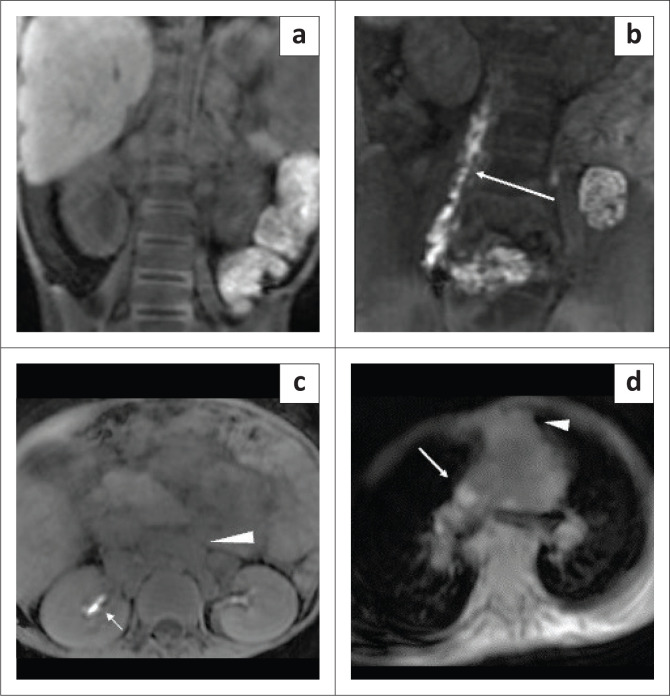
Dynamic contrast enhanced MR lymphangiography was performed in the same child for chylous ascites. (a) Coronal pre-contrast T1-weighted image of the abdomen and pelvis and (b) coronal T1-weighted image of the abdomen and pelvis at 10 min after nodal contrast injection showed dilated lumbar lymphatics (arrow) and non-opacification of the central conducting lymphatics. (c) Axial T1-weighted image of the abdomen 30 min after intranodal contrast administration showed non-opacification of the central conducting lymphatics. Contrast opacification was noted in the renal pelvis. Enlarged abdominal lymph nodes could also be seen (arrow head). (d) Axial T1-weighted image of the chest 40 min after intranodal contrast administration showed non-opacification of the thoracic duct in the mediastinum but contrast opacification was visible in the superior vena cava (arrow). Enlarged lymph nodes were seen in the anterior mediastinum (arrow head).
